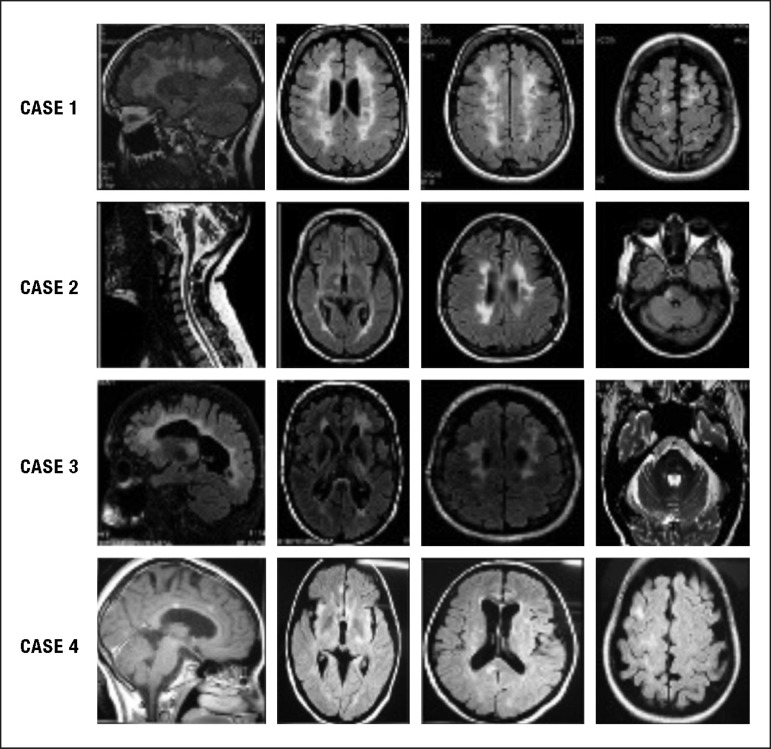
MRI of the four MS patients. Severe brain atrophy and basal ganglia lesions are evident in all four
